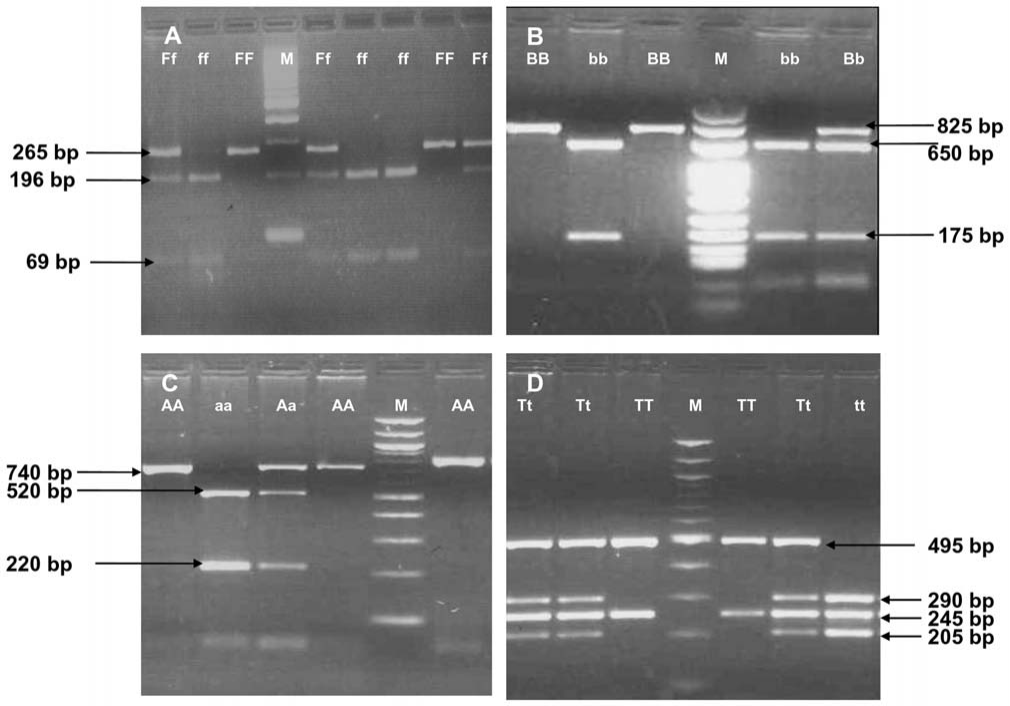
The genotypes for the four SNPs were determined by PCR amplification and restriction digestion of the PCR products with enzymes *FokI, BsmI, ApaI*, and *TaqI*. A: Fok1 digestion: SNP C/T in exon 2 was studied by amplifying a 265 bp fragment using primers 5′AGCTGGCCCTGGCACTGACTCTTGCTCT 3′ and 5′ATGGAAACACCTTGCTTCTTCTCCCTC 3′ with 68°C as annealing temperature and digestion by fok1 at 37°C for 3 hours. Presence of restriction site is denoted by ‘f’ while absence of restriction is denoted by ‘F’. Results show FF (CC) i.e., a 265 bp band or Ff (CT) i.e., 265 bp, 196 bp and 69 bp, bands, ff (TT) i.e., 196 bp and 69 bp bands. M is the100 bp ladder. B: Bsm 1 digestion: SNP A/G in Intron 8 was studied by amplifying an 825 bp fragment using primers 5′CAACCAAGACTACAAGTACCGCGTCAGTGA 3′ and 5′AACCAGCGGGAAGAGGTCAAGGG 3′ with 65°C as annealing temperature and digestion by Bsm1 at 65°C for one hour. Presence of restriction site is denoted as ‘b’ and absence of restriction is denoted by ‘B’. The results show BB (AA) i.e., 825 bp band, Bb (AG) i.e., 825 bp, 650 bp and 175 bp and bands and bb (GG) 650 bp and 175 bp bands. M is 25 bp ladder. C: Apa 1 digestion: SNP T/G in Intron 8 was studied by amplifying an 740 bp fragment using primers 5′ CAGAGCATGGACAGGGAGCAAG 3′ and 5′ GCAACTCCTCATGGCTGAGGTCTCA 3′ with 65°C as annealing temperature and digestion by Apa1 at 37°C for one hour. Presence of restriction site is denoted as ‘a; and absence of restriction is denoted by ‘A’. The results show AA (TT) i.e., 740 bp band, Aa (TG) i.e., 740 bp, 520 bp and 220 bp bands and aa (GG) 520 bp and 220 bp bands. M is 100 bp ladder. D: Taq 1 digestion: SNP T/C in exon 9 was studied by amplifying an 740 bp fragment using primers 5′CAGAGCATGGACAGGGAGCAAG 3′ and 5′GCAACTCCTCATGGCTGAGGTCTCA 3′ with 65°C as annealing temperature and digestion by Taq1 at 65°C for one hour. Presence of restriction site is denoted as ‘t’ and absence of restriction is denoted by ‘T’. The results show TT (TT) i.e., 495 bp and 245 bp bands Tt (TC) i.e., 495 bp, 290 bp, 245 bp and 205 bp bands and tt (CC) 290 bp, 245 bp and 205 bp bands. M is 100 bp ladder.
